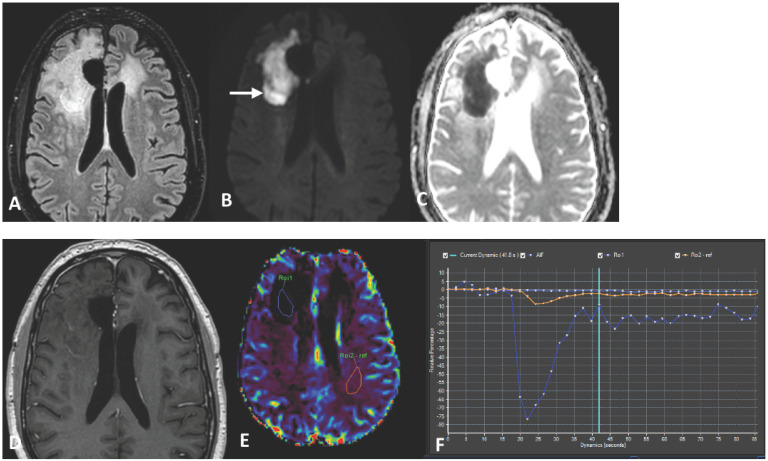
The last MRI, performed fifteen months after diagnosis, shows less edema and an improvement in the mass effect (A). Marked DWI restriction persists (B) with very low ADC values (C). There is a slight growth in the lesion from its posterior margin (white arrow in (B)). Peripheral enhancement has decreased on the T1 CE sequence (D). DSC perfusion imaging shows decreased rVSC compared to the normal contralateral white matter (E), with an almost flat dynamic curve (light blue in (F)). Persistent marked DWI restriction, but contrast enhancement improvement, and decreased perfusion suggest a mixture of mechanisms, partly radionecrosis with some degree of superimposed pseudoprogression, due to regorafenib.
